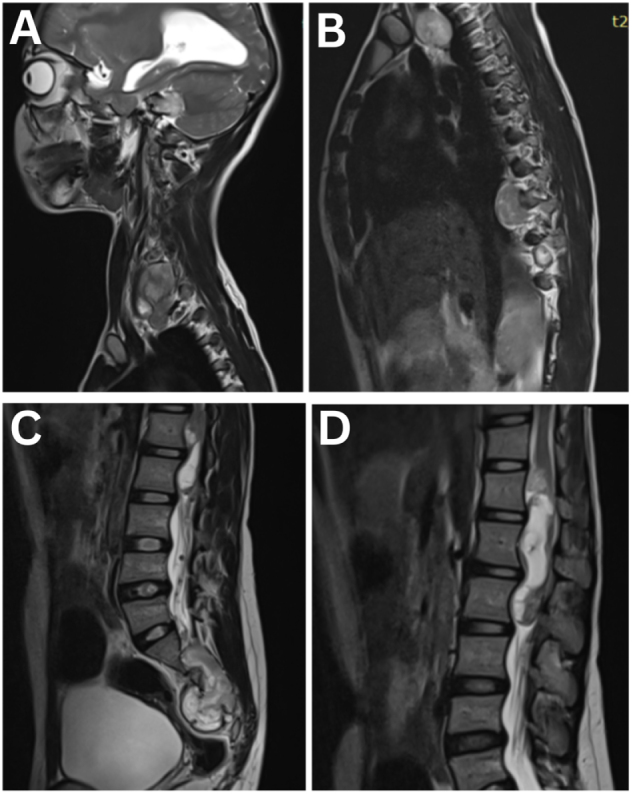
(A) Sagittal T2-weighted MRI of the cervical spine demonstrating a lobulated, heterogeneous lesion extending into the neural foramina along the exiting nerve roots, with features suggestive of a nerve sheath tumor and extramedullary intradural extension at the C6–D1 level. (B) Sagittal T2-weighted MRI of the dorsal spine showing similar lobulated lesions with intradural extramedullary extension at the D9–D10 level. (C) Sagittal T2-weighted MRI of the sacrococcygeal spine demonstrating extension of the lesion into the neural foramina at the sacrococcygeal level. (D) Sagittal T2-weighted MRI of the lumbosacral spine showing an intramedullary lesion involving the terminal spinal cord and filum terminale, extending from D12 to L2, resulting in fusiform dilatation of the cord and scalloping of the posterior vertebral margins.
